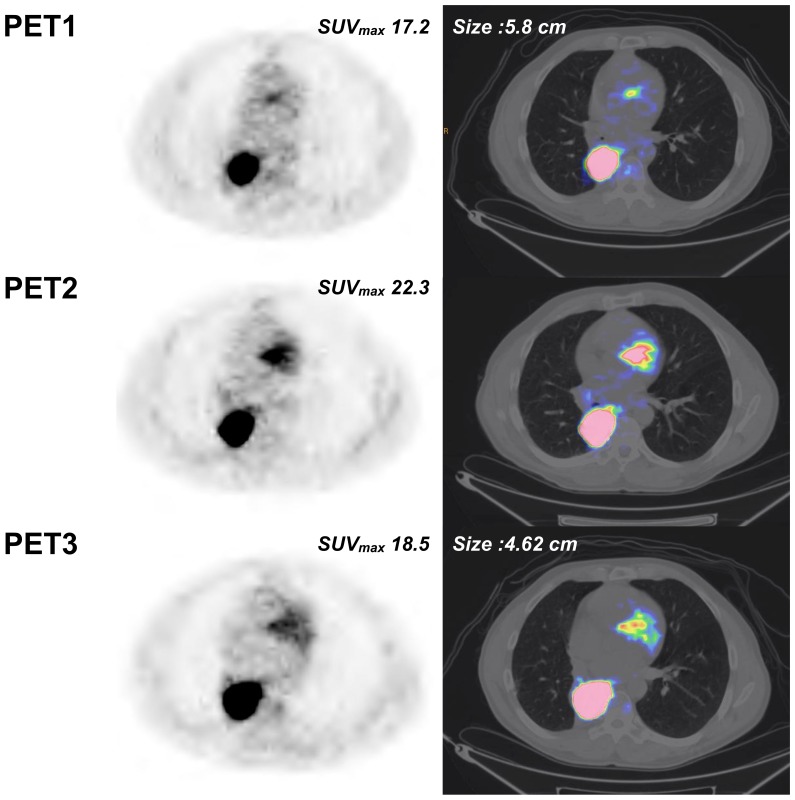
Example of a patient with discordant PET2 and conventional imaging. Patient with right upper lobe NSCLC associated with subcarinal lymphadenopathy and ipsilateral lung metastasis (patient #9). Sum of the SUVmax of the most hypermetabolic lesions (2 lung lesions, 1 mediastinal lymph node) were 25.2, 29.3 (+16.3%) and 23.8 (−5.4%) for PET1, PET2 (% versus PET1) and PET3 (% versus PET1), respectively. Based on a SUVmax cut-off value of −21.6, the patient was classified as mP on PET2, in contrast with RECIST evaluation on CT scan (performed 71 days after starting erlotinib). This patient was subsequently reclassified as mNP on PET3 in accordance with RECIST evaluation with a 5.4% decrease of SUVmax (cut-off: 18.5%).
